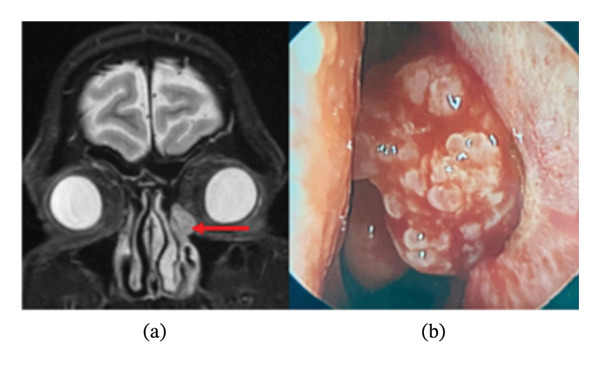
(a) Coronal T2‐weighted MRI of the orbits with IV contrast demonstrating a 1.1 × 1.1 × 1.5 cm enhancing lesion in the left lacrimal sac, with no evidence of bony erosion (red arrow). (b) Intraoperative nasal endoscopy of the mass following incision of the lacrimal sac.
